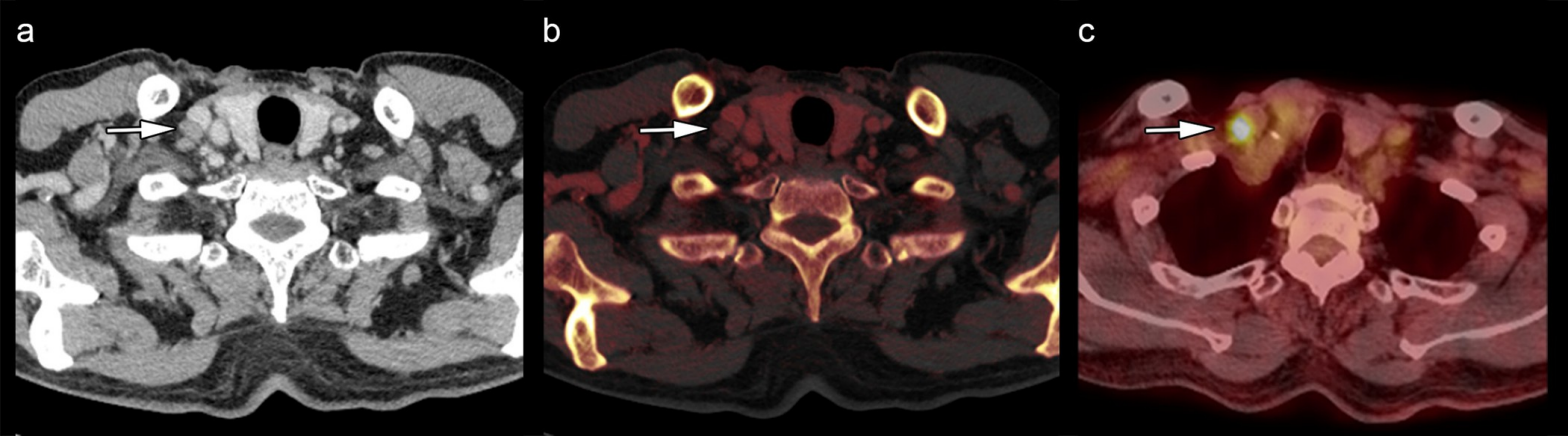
Representative dual-energy CT and PET images in patients with lymph node metastasis. Contrast-enhanced CT image (a), iodine map (b), and PET image (c) of an 83-year-old man with esophageal cancer; images show swelling of the right supraclavicular lymph node (a, b, c arrows), which was confirmed as metastasis postoperatively. The low iodine concentration (1.45 mg/mL) and high maximal standardized uptake value (10.7) were measured. CT: computed tomography; PET: positron-emission tomography.
